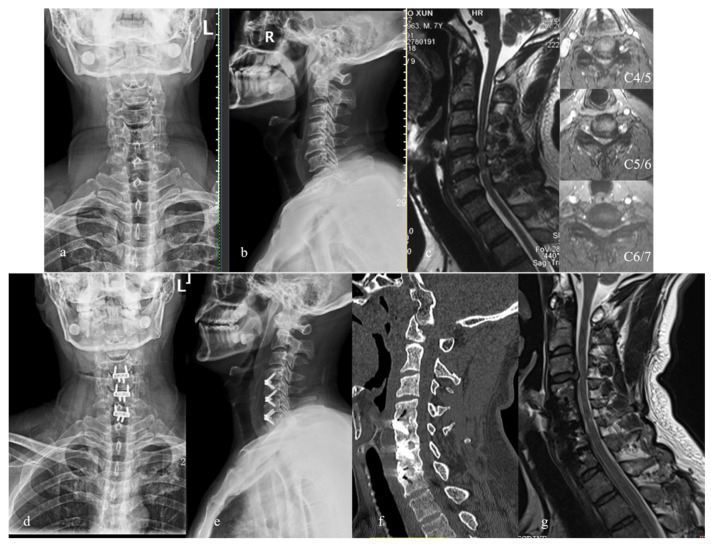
A 54-year-old male with walking disturbance and clumsy hands for 5 years and radiating pain in the left arm for 6 months. (a,b) Preoperative x-ray films; (c) preoperative MRI showing C4-C7 disc herniation compressing the spinal cord, and stenosis on the left nerve root canals; (d,e) postoperative X-ray films showing C4-C7 ACDF with Zero-P spacers; (f) CT scan at 2-year follow-up showing good implant position and bone fusion; (g) MRI at 2-year follow-up showing ASD at C3/4; however, there was no complaint of any symptoms and observation was implemented.
